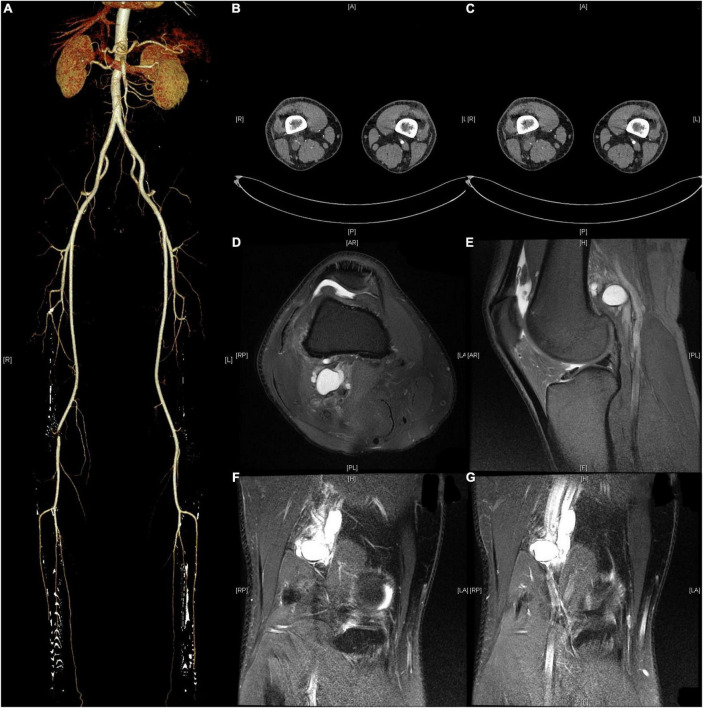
Preoperative CTA and MRI. (A–C) CTA showed severe stenosis of the popliteal artery in the right lower extremity. (D–G) MRI suggested that the popliteal artery was compressed by an oval cyst with high signal intensity on T2-weighted, which is 1.7*2.5*3.1 cm in volume.
